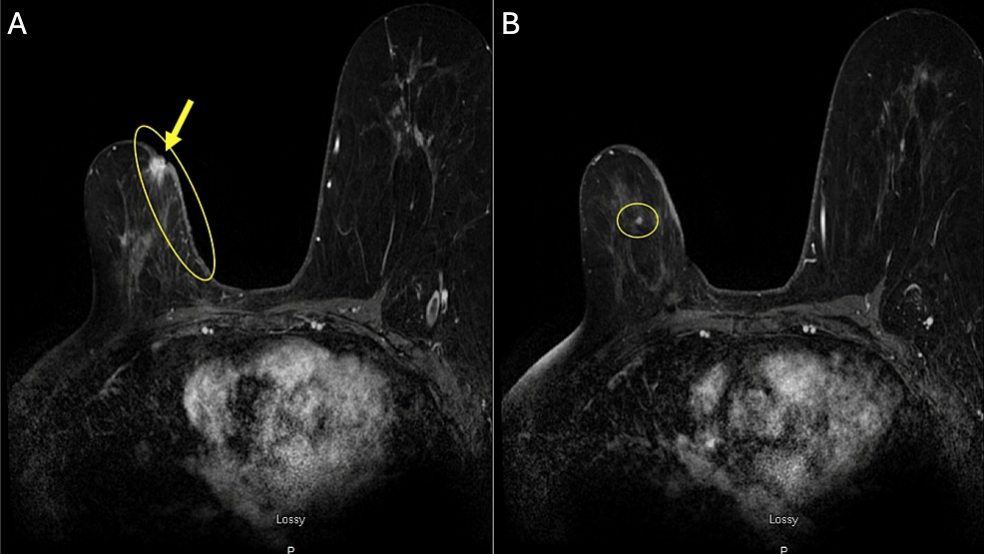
Axial T1 post-contrast MRI images of the right breast demonstrating skin thickening (panel A, ovoid) with associated enhancement of the nipple-areolar complex (panel A, arrow). Panel B shows a mass at 12 o'clock, measuring up to 5 mm.
